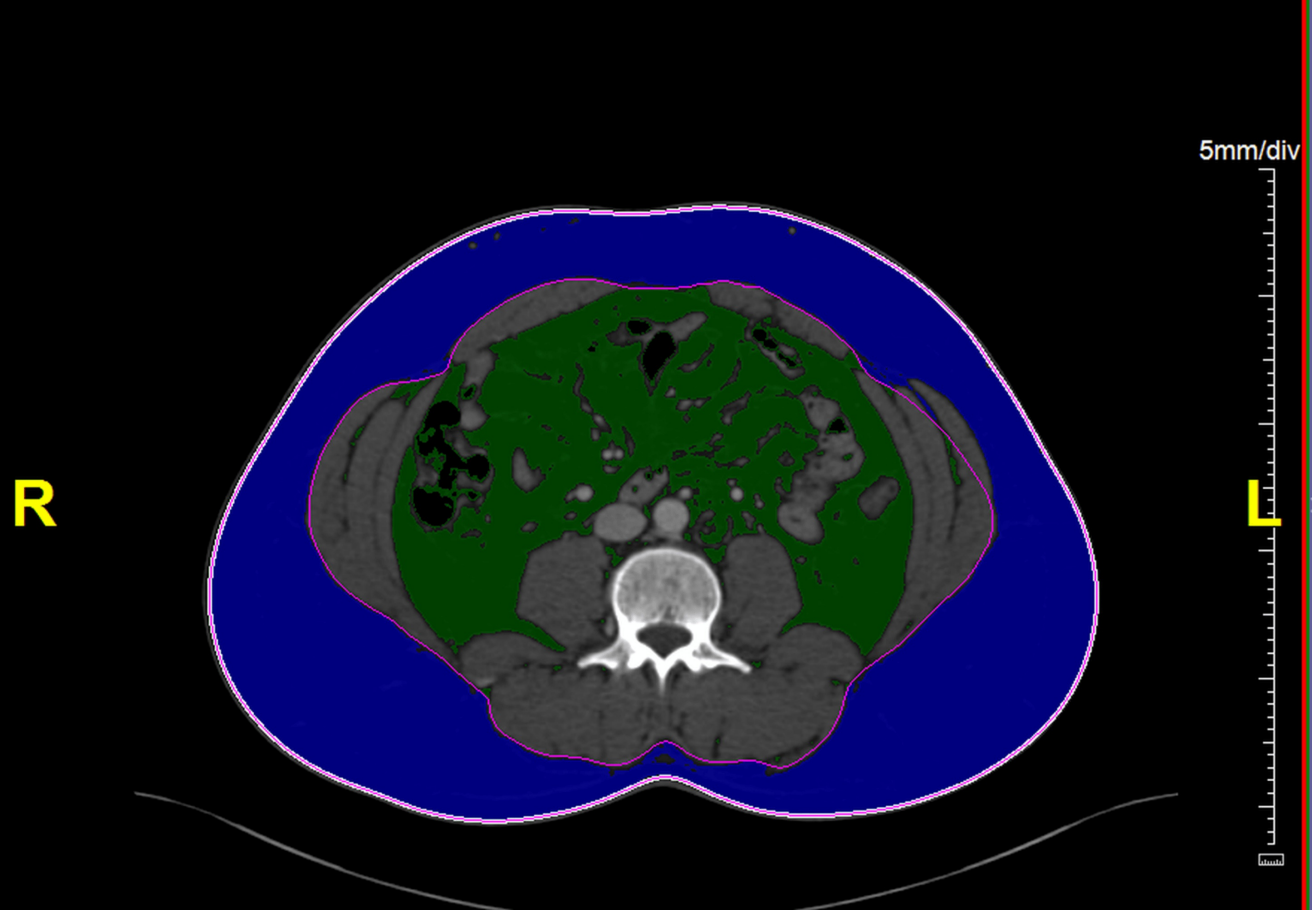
CT scan at the mid-L3 level showing subcutaneous fat (blue) and visceral fat (green) areas In this example, visceral fat measures 140 cm², and subcutaneous fat measures 286 cm². Adipose tissue was identified using CT HU values of -190 to -30 HU. L3, third lumbar vertebra
